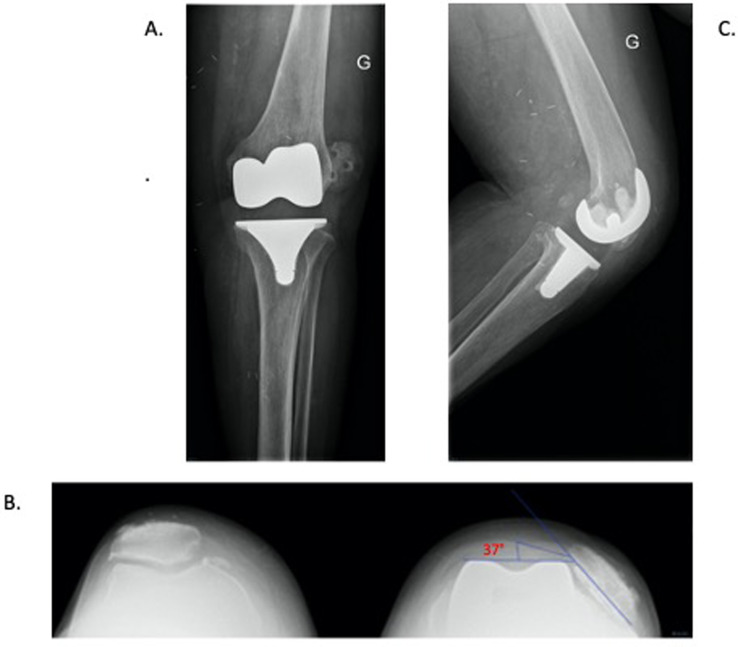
Pre-MPFL reconstruction. Pre-operative X-rays of a patient with lateral patella dislocation post total knee arthroplasty. (A) AP X-ray. The patella is seen dislocated laterally. (B) Sky-line view demonstrating patella dislocation and patella-tilt of 37°. (C) Lateral profile X-ray.
